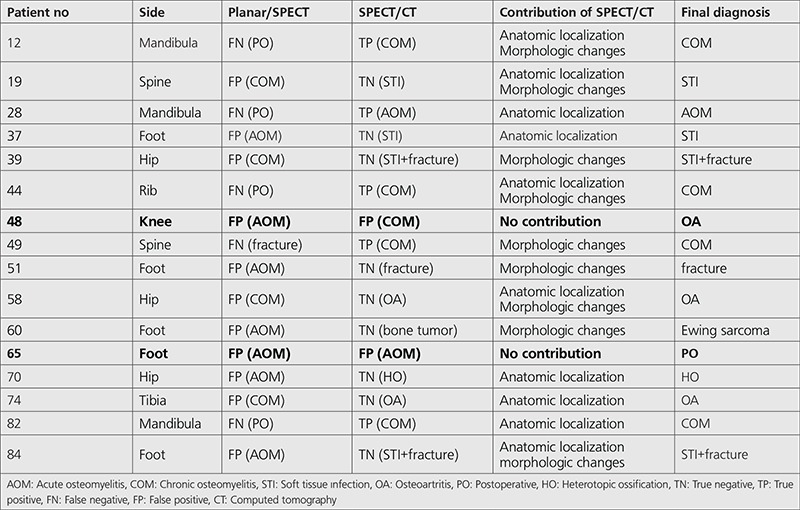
The contribution of single photon emission computed tomography (SPECT)/CT in the patients who had false negative and false positive results
